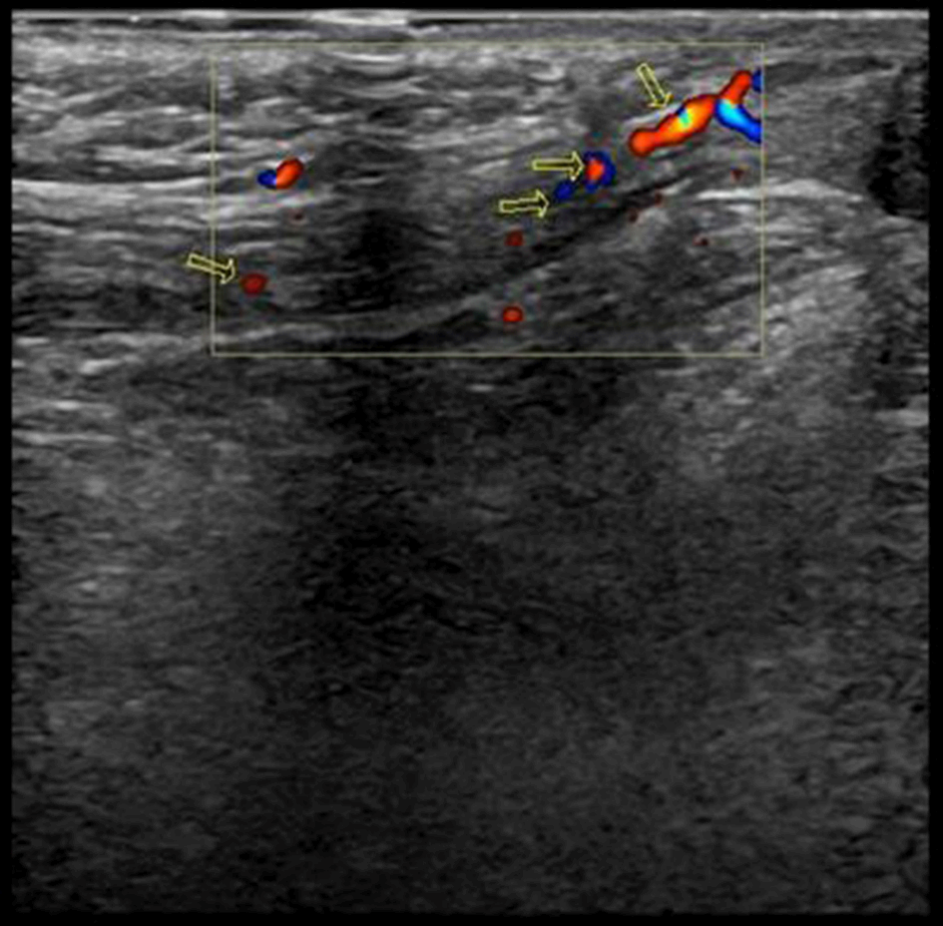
Color Doppler Flow Imaging (CDFI) showed the flow signal.
